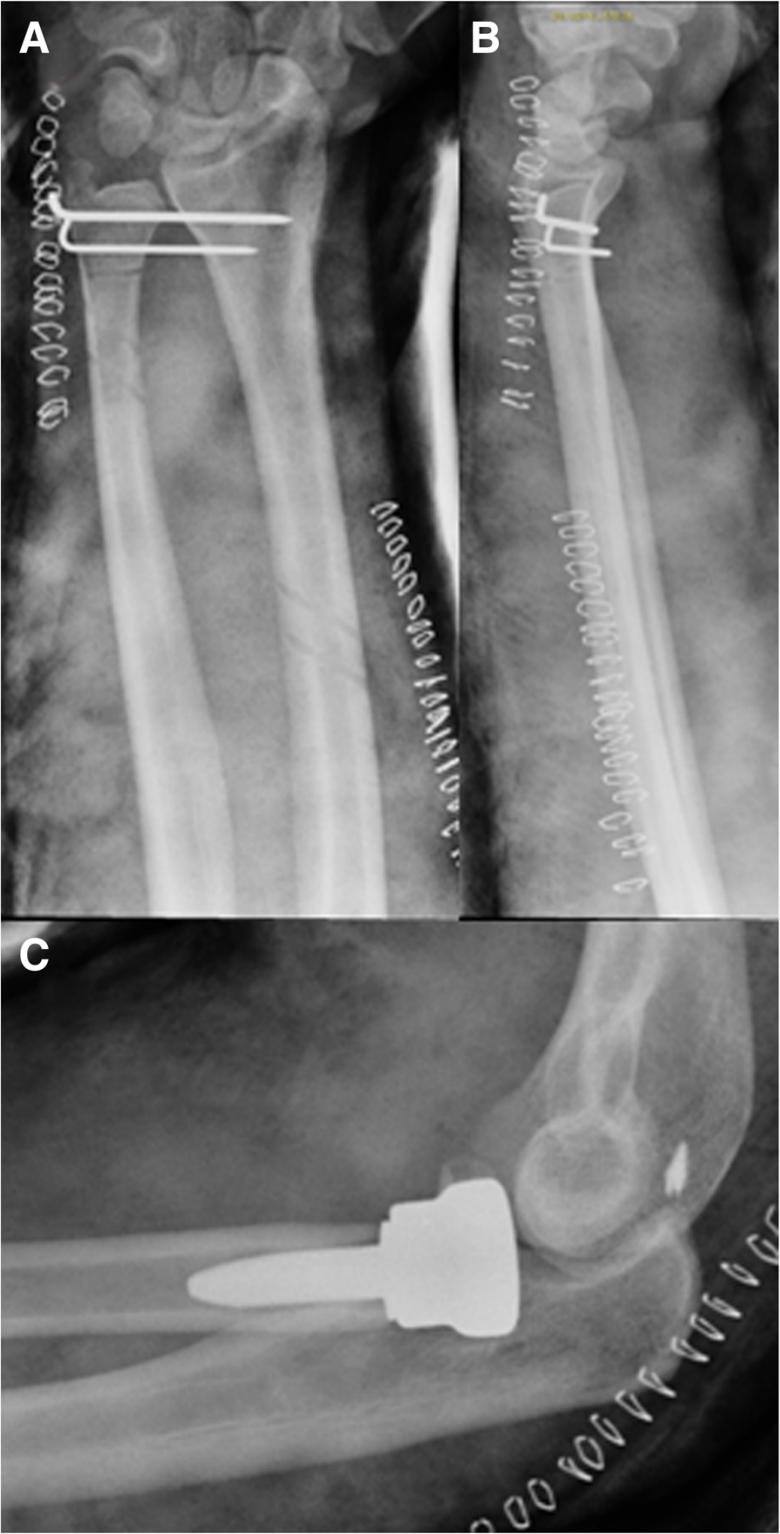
Post operative X-rays, clinical case 3. Post operative X-rays show the reduced and stabilized DRUJ (a, b) and the radial head prosthesis (c). It is possible to see the radial and ulnar tunnels of the two bundles of the newly reconstructed IOM (a)
